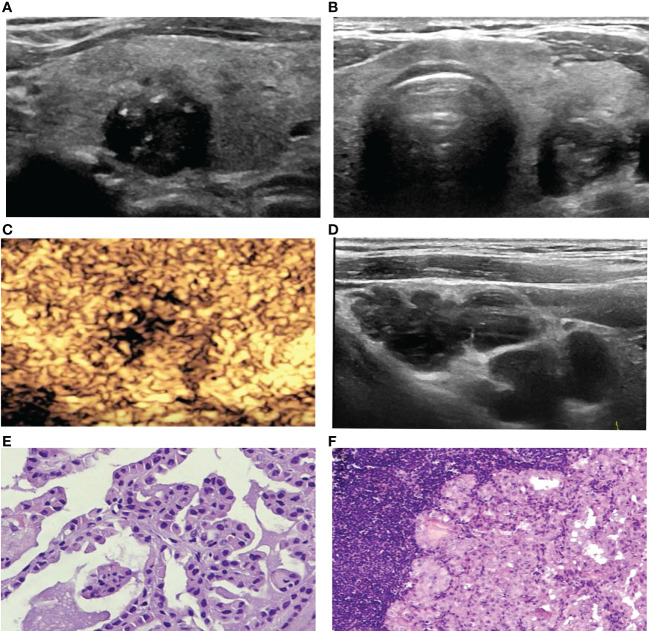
A 67-year-old woman with PTC. (A) Preoperative ultrasound images showing that the size of the tumor was approximately 1.2*1.0*1.3 cm in the left lobe, the margin was irregular, taller than wide, and calcification was detected. (B) Transverse sonogram showing that the contact range with the capsule was approximately 25%-50%. (C) CEUS showing heterogeneous hypoenhancement in the nodule. (D) Metastatic lymph node showing that the normal structure disappeared, presenting cystic changes. (E) Surgical pathology confirming PTC (HE, original magnification, ×100). (F) Surgical pathology confirming cervical lymph node metastasis from PTC, (HE, original magnification, ×100).
